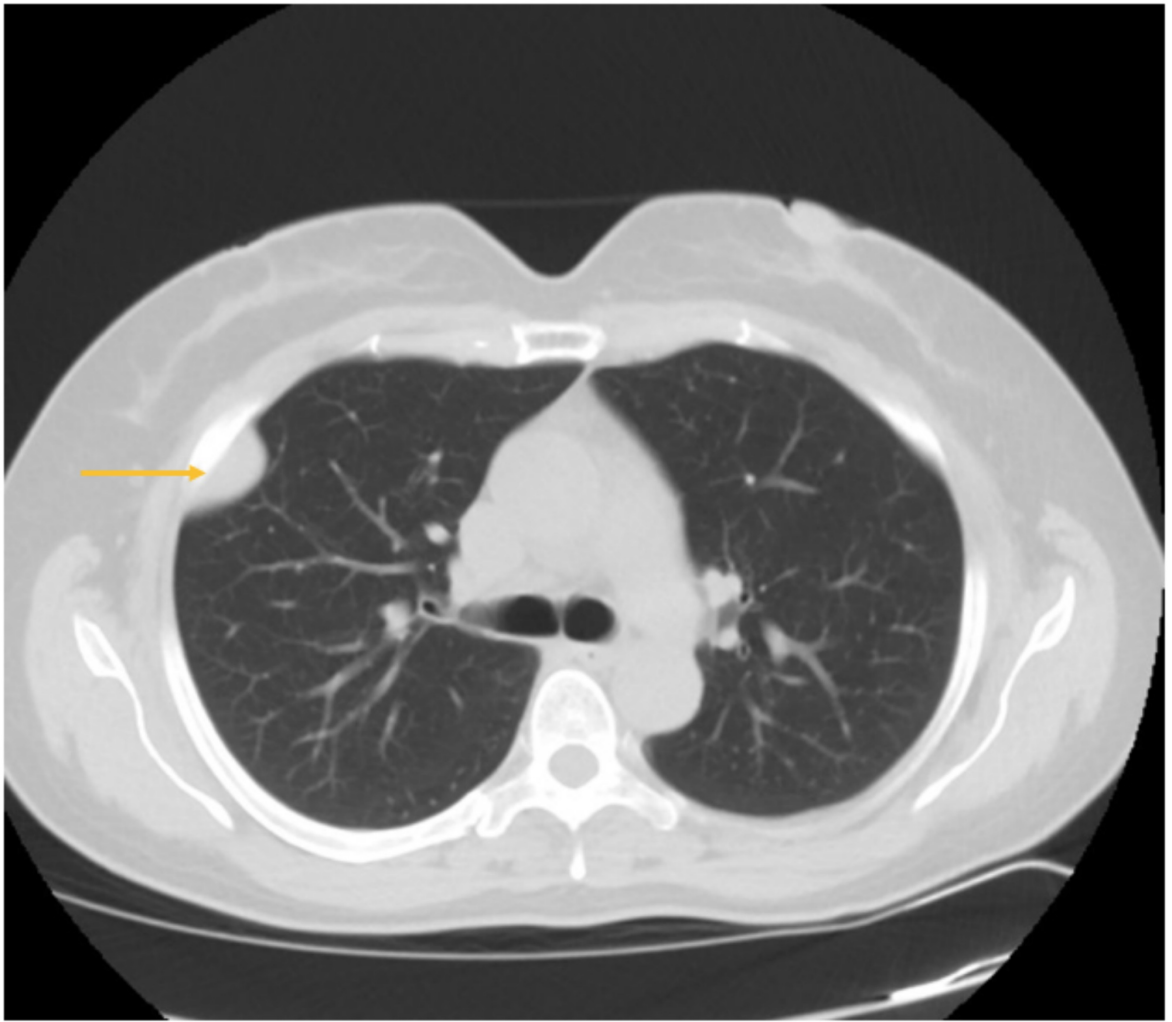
CT scan showing solitary fibrous pleural tumor.
